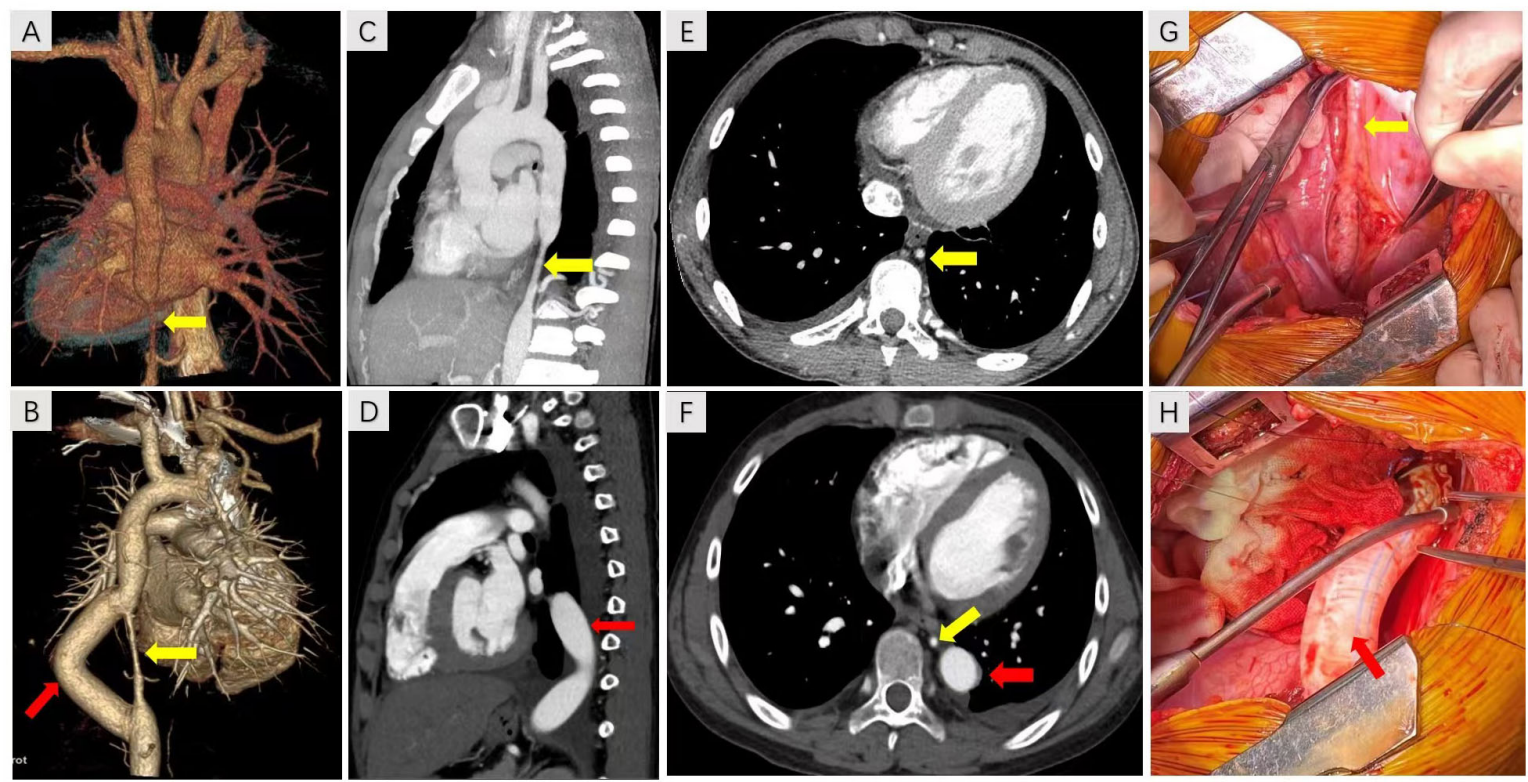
The CTA images of thorax before and after surgery. (A,B) The 3D reconstruction image of the stenosis (yellow arrow) and GORE-TEX Vascular Graft (red arrow). (C–F) The descending aortic stenosis (yellow arrow) and GORE-TEX Vascular Graft (red arrow) were visualized in the thoracic CT before and after surgery. (G) The stenosis of descending aorta. (H) A GORE-TEX Vascular Graft was used to establish a vascular bypass.
